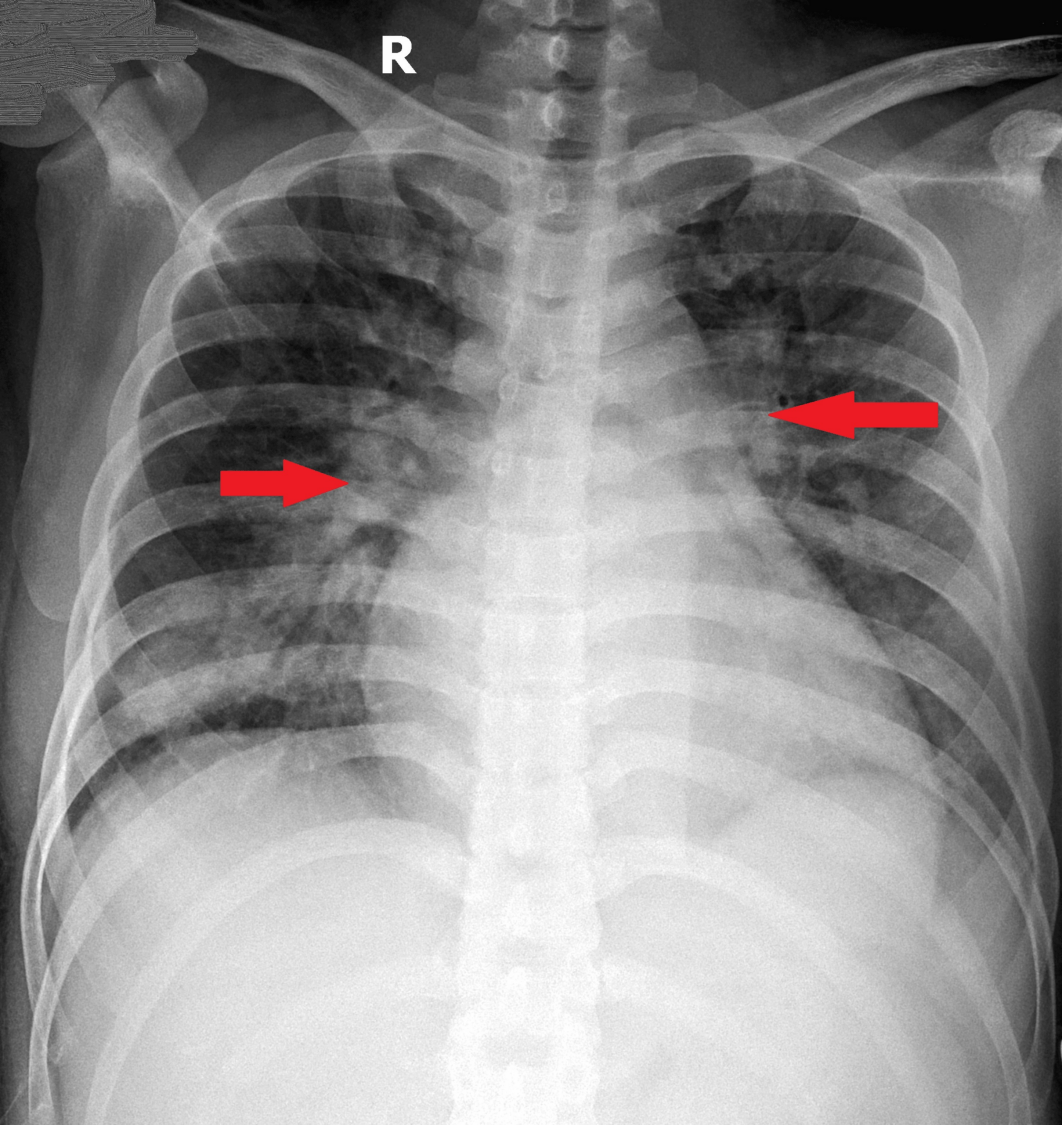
Chest X-ray showing bilateral perihilar consolidations (red arrows)
